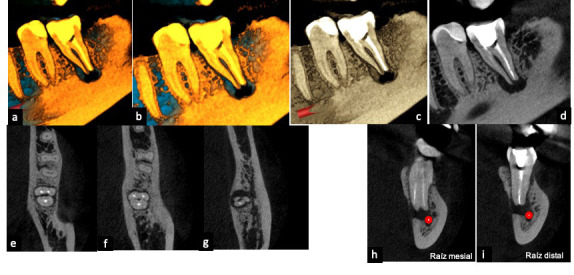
Imágenes de CT: a) y b) Renderizado 3D; c) y d) Corte sagital; e), f) y g) Cortes axiales; h) e i) Cortes coronales. Cortes de tomografía cone-beam de diente 37, para seguimiento de los resultados del tratamiento.
